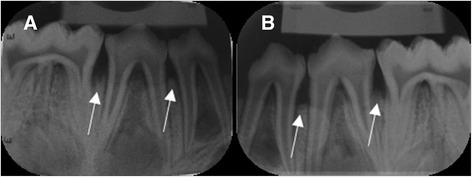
Periapical radiographs. a. Six-hour lesion between first molar and second premolar. No lesion (control) between first and second premolar. b. Four-hour lesion between first and second premolar. Two-hour lesion between second premolar and first molar
